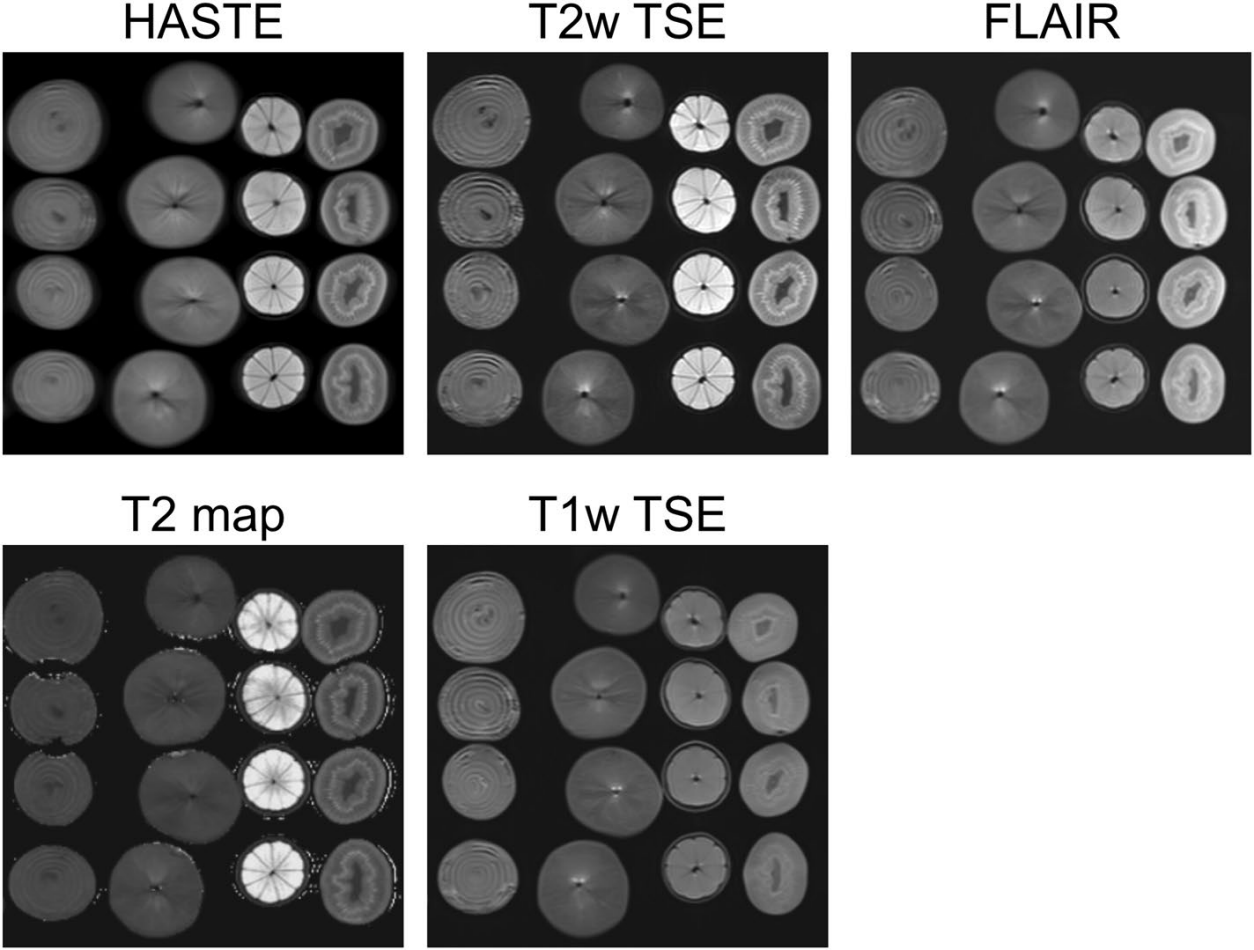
Representative images of the acquired magnetic resonance imaging sequences. FLAIR, fluid-attenuated inversion recovery; HASTE, half-Fourier acquisition single-shot turbo spin-echo; T1w, T1-weighted; T2w, T2-weighted; TSE, turbo-spin-echo.
